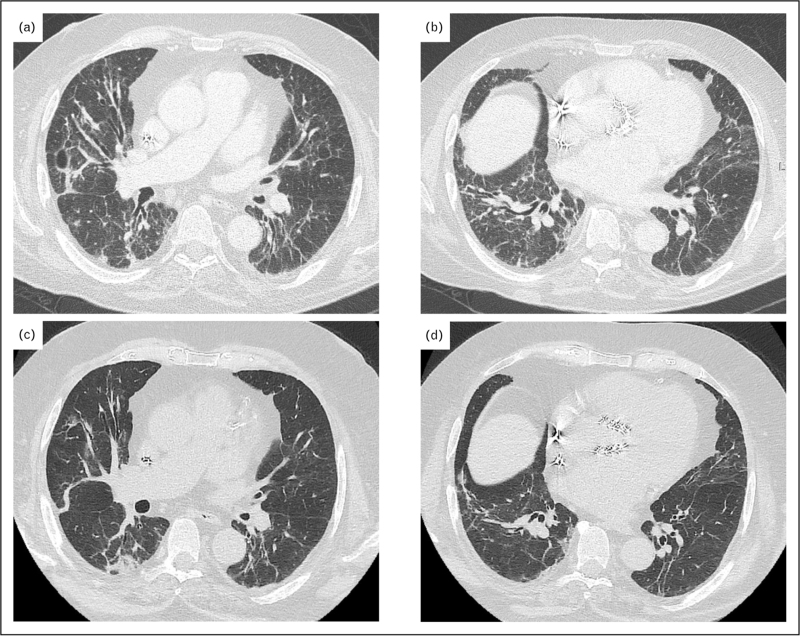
Organizing pneumonia/nonspecific interstitial pneumonia pattern in a 79-year-old man undergoing nivolumab therapy for advanced melanoma. (a, b) Axial chest computed tomography images obtained 3 months after nivolumab therapy show multifocal mid- and lower-lung linear opacities and airspace consolidations, a finding consistent with an organizing pneumonia pattern of pneumonitis. (c, d) Axial chest computed tomography images obtained 2 years later after withholding nivolumab and administering systemic steroid therapy show significant residual disease.
